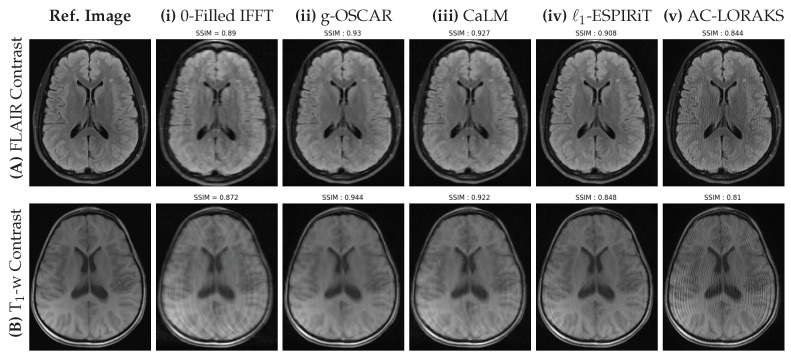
Retrospective results for a single slice of FLAIR (top row) and T1 weighted (bottom row) images of the brain fastMRI data set obtained using the Cartesian mask shown in Figure 3A with UF=4, corresponding to AF≃4. The fully sampled Cartesian reference and the different methods (Zero filled Inverse, g-OSCAR, CaLM, L1-ESPiRIT and AC-LORAKS) are shown from left to right and the SSIM scores are indicated to reflect the performance of each method.
